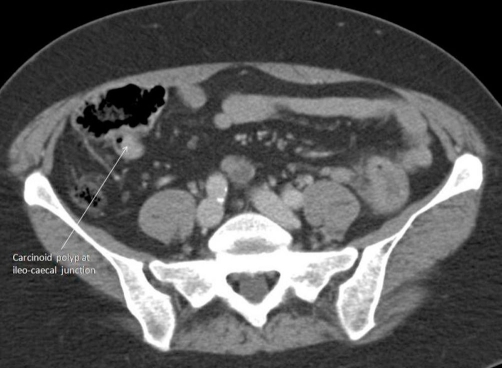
CT scan image showing polypoidal lesion at ileocaecal junction.
